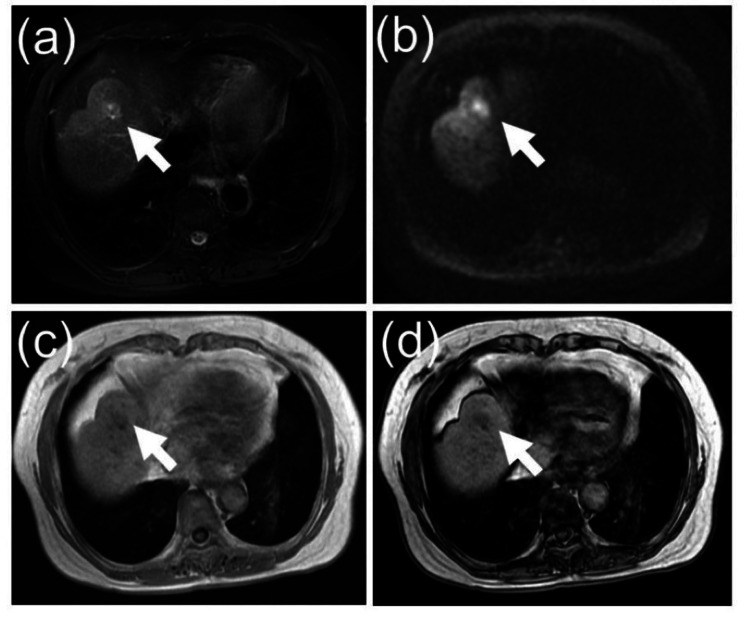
Non-contrast MRI images of recurrent hepatocellular carcinoma There is a 17 mm hepatocellular carcinoma (white arrow) in segment IV of the liver, which shows high signal intensity on fat-suppressed T2-weighted images and diffusion-weighted images, with the signal decreasing from in-phase to out-of-phase. (a) Fat-sat T2-weighted image; (b) Diffusion-weighted image; (c) T1-weighted in-phase image; (d) T1-weighted out-of-phase image.
